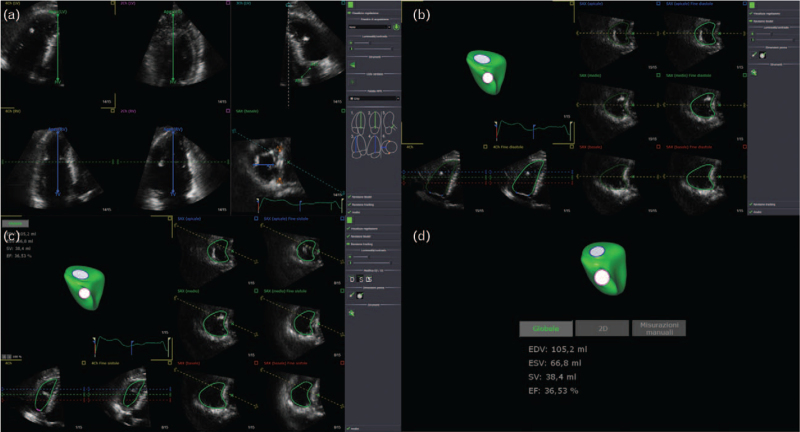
Phases of the postprocessing of echocardiographic images with GE EchoPAC software. (a) Finding landmarks with 4D RV function. (b) ‘Beutel revision phase’: the endocardial edge detection of the RV in the end-diastolic phase. (c) ‘Tracking revision phase’: the intracavitary perimeter was identified by the software during the entire cardiac cycle. (d) RV parameters and 3-D reconstruction. RV, right ventricle.
